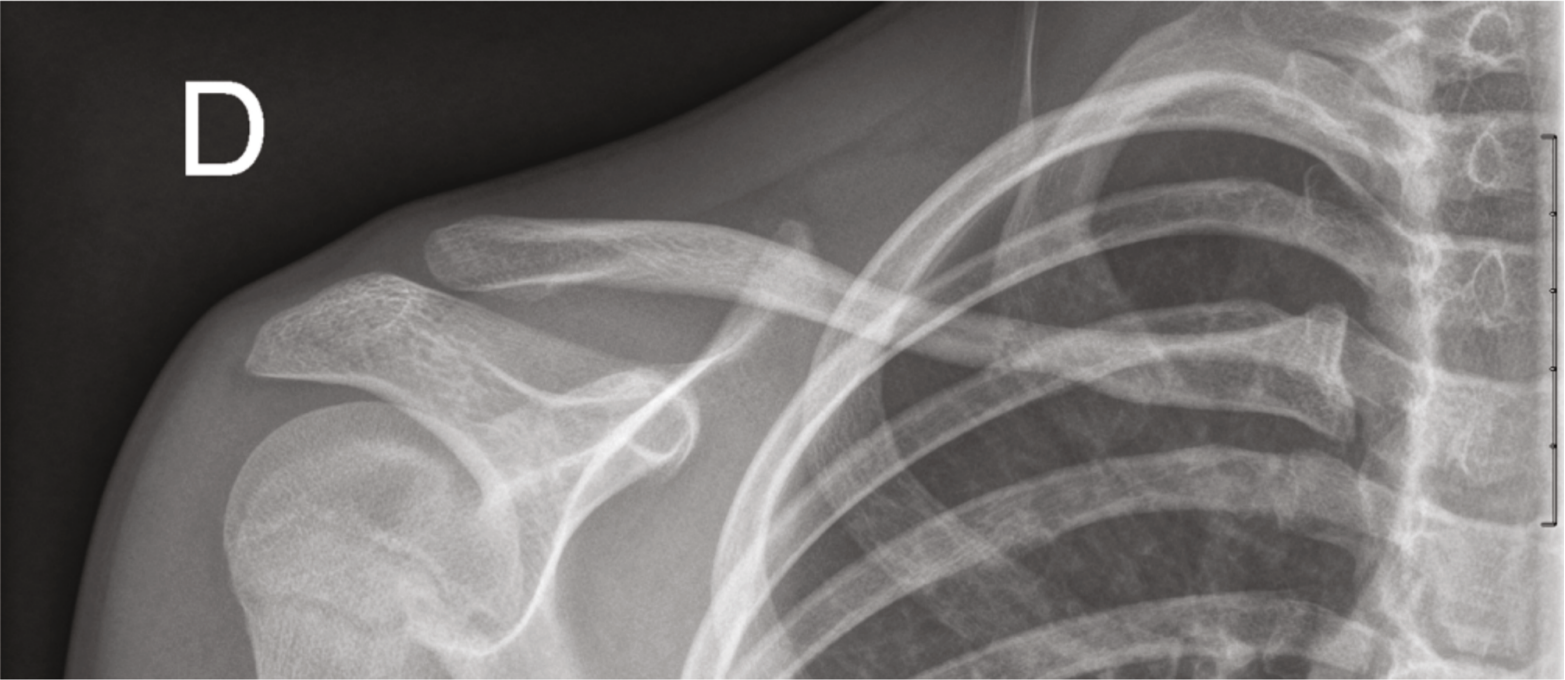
X-ray imaging showing the nondislocated fracture on the day of presentation to the paediatric A&E.
